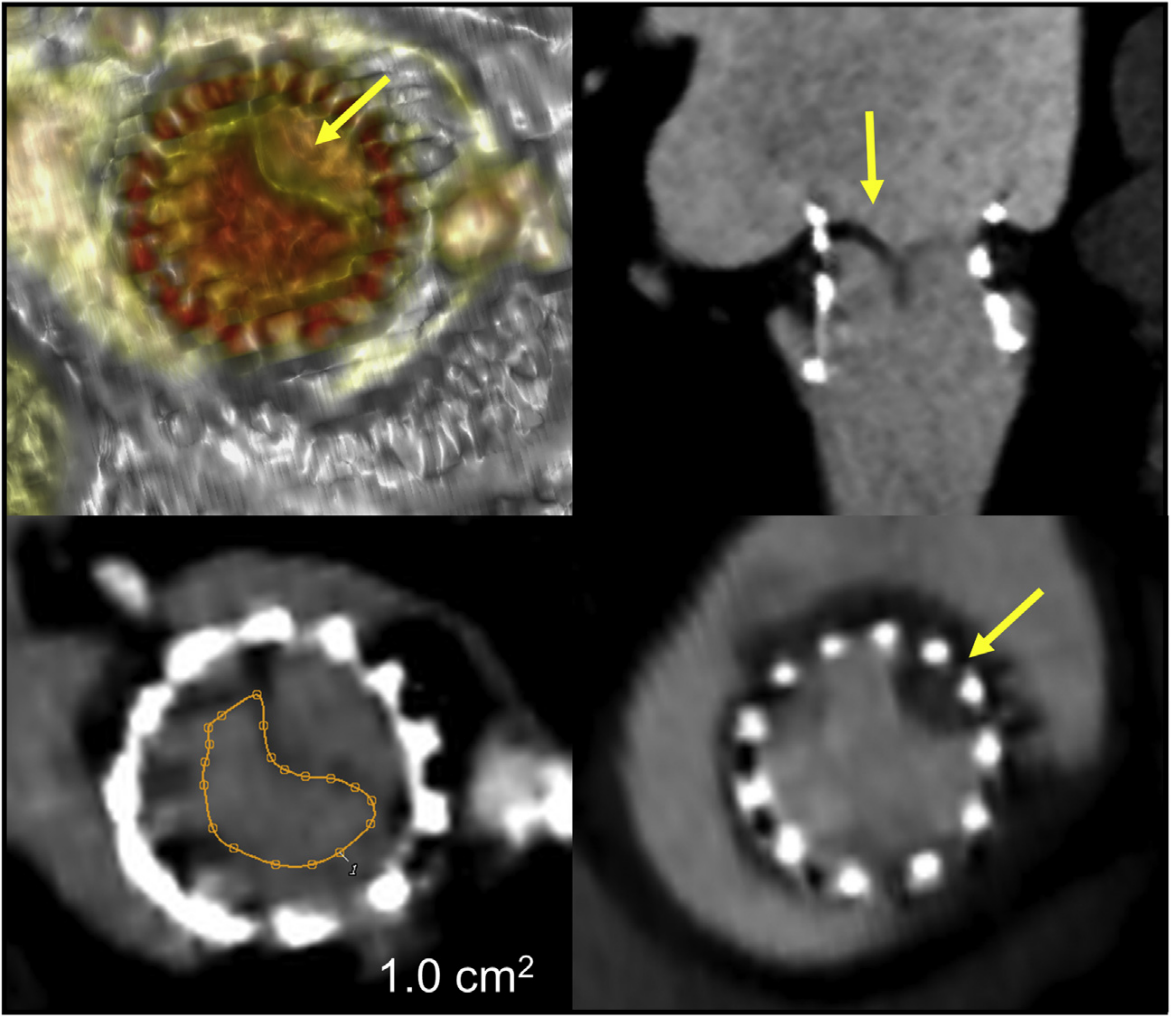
Evaluation of prosthesis leaflet mobility after transcatheter mitral valve replacement by MDCT. A patient who received ViV TMVR presented with elevated transmitral gradient of 7 mm ​Hg seen on 1 mo post-TMVR echocardiography study. Hypoattenuating leaflet thickening (HALT) with restricted mobility of the 1 leaflet (yellow arrow) is seen on functional MDCT. The THV opening area is as small as 1.0 cm^2^ (orange tracing).
